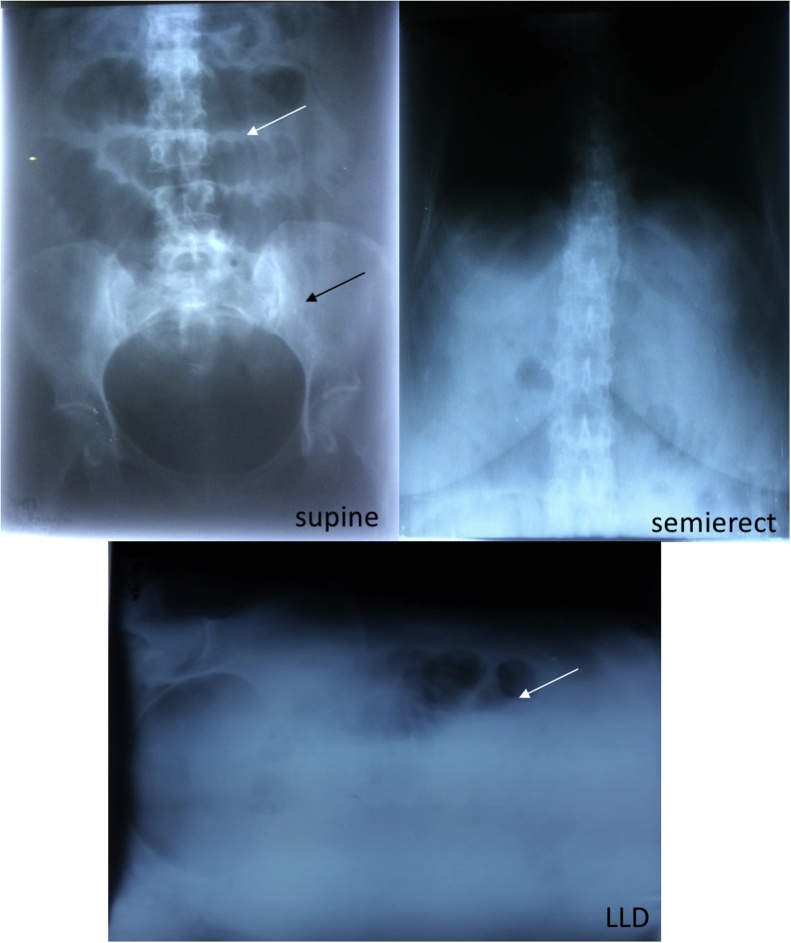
Abdominal X-ray revealed findings of small bowel obstruction. Supine view demonstrated dilatation of multiple loops of small bowel (white arrow). Large bowel was collapsed and absent of air in the rectosigmoid (black arrow). At the lateral left decubitus (LLD), multiple air-fluid levels were shown in the dilated loops of small bowel obstruction (white arrow).
